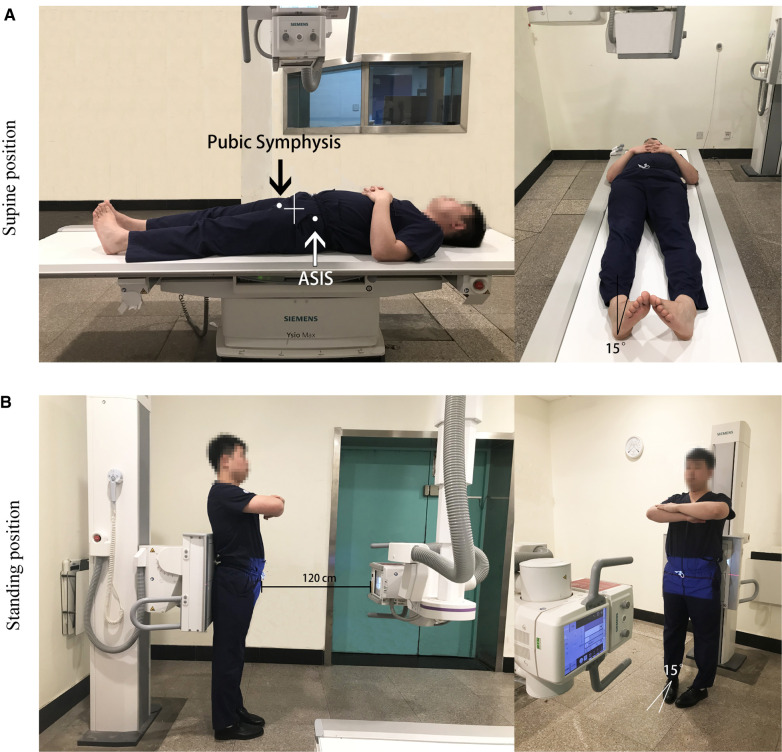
Standard body positions of AP pelvic radiographs. (A) The AP pelvic radiographs of supine position. (B) The AP pelvic radiographs of standing position. ASIS is anterior superior iliac spine.
